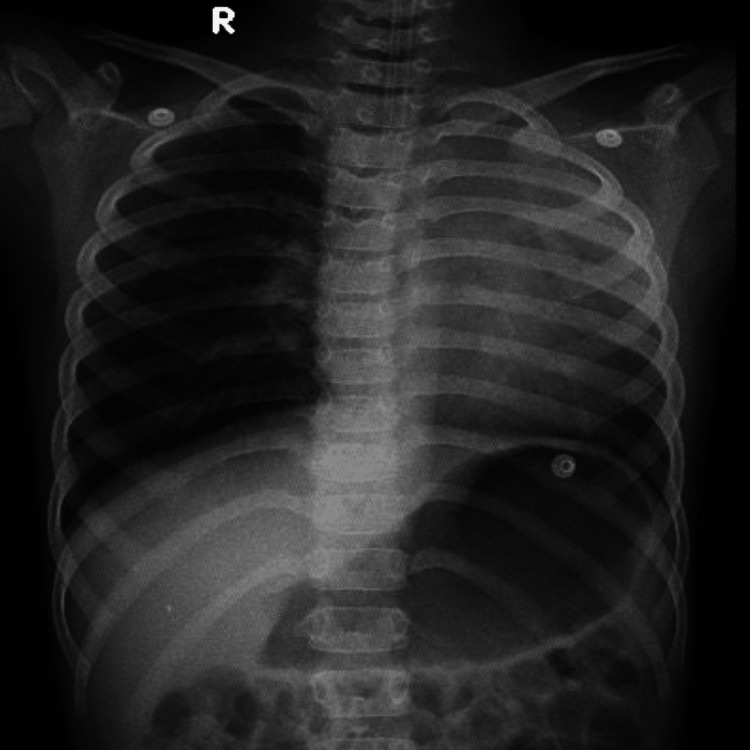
Post-procedure Chest Radiograph Demonstrating Re-expansion of the Left Lung Follow-up chest radiograph demonstrating interval improvement in left lung aeration and re-expansion following successful retrieval of the aspirated toy whistle components.
